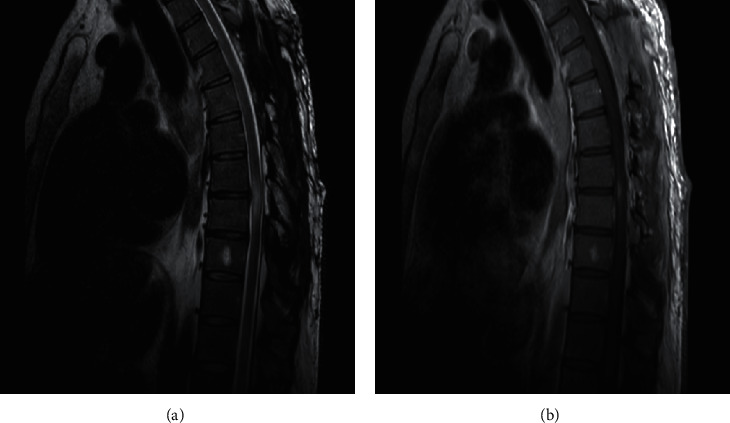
(a) Sagittal T2 MRI of whole spine after 2 months of treatment with mycophenolate mofetil showing decrease in T2 signal involving T7-T8. (b) Sagittal T1 MRI with gadolinium after 2 months of treatment showing further decrease in extent of enhancing lesion.
